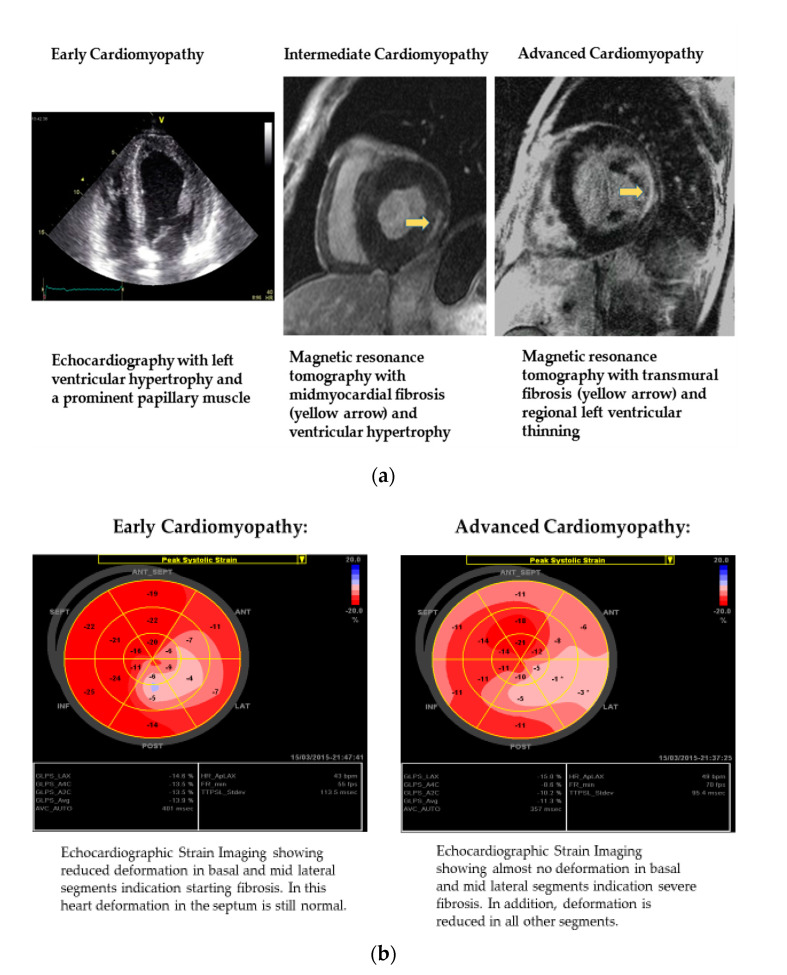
(a) Echocardiographic aspects and magnetic resonance tomography findings in various stages of Fabry cardiomyopathy. (b) Strain analysis in various stages of Fabry cardiomyopathy.
